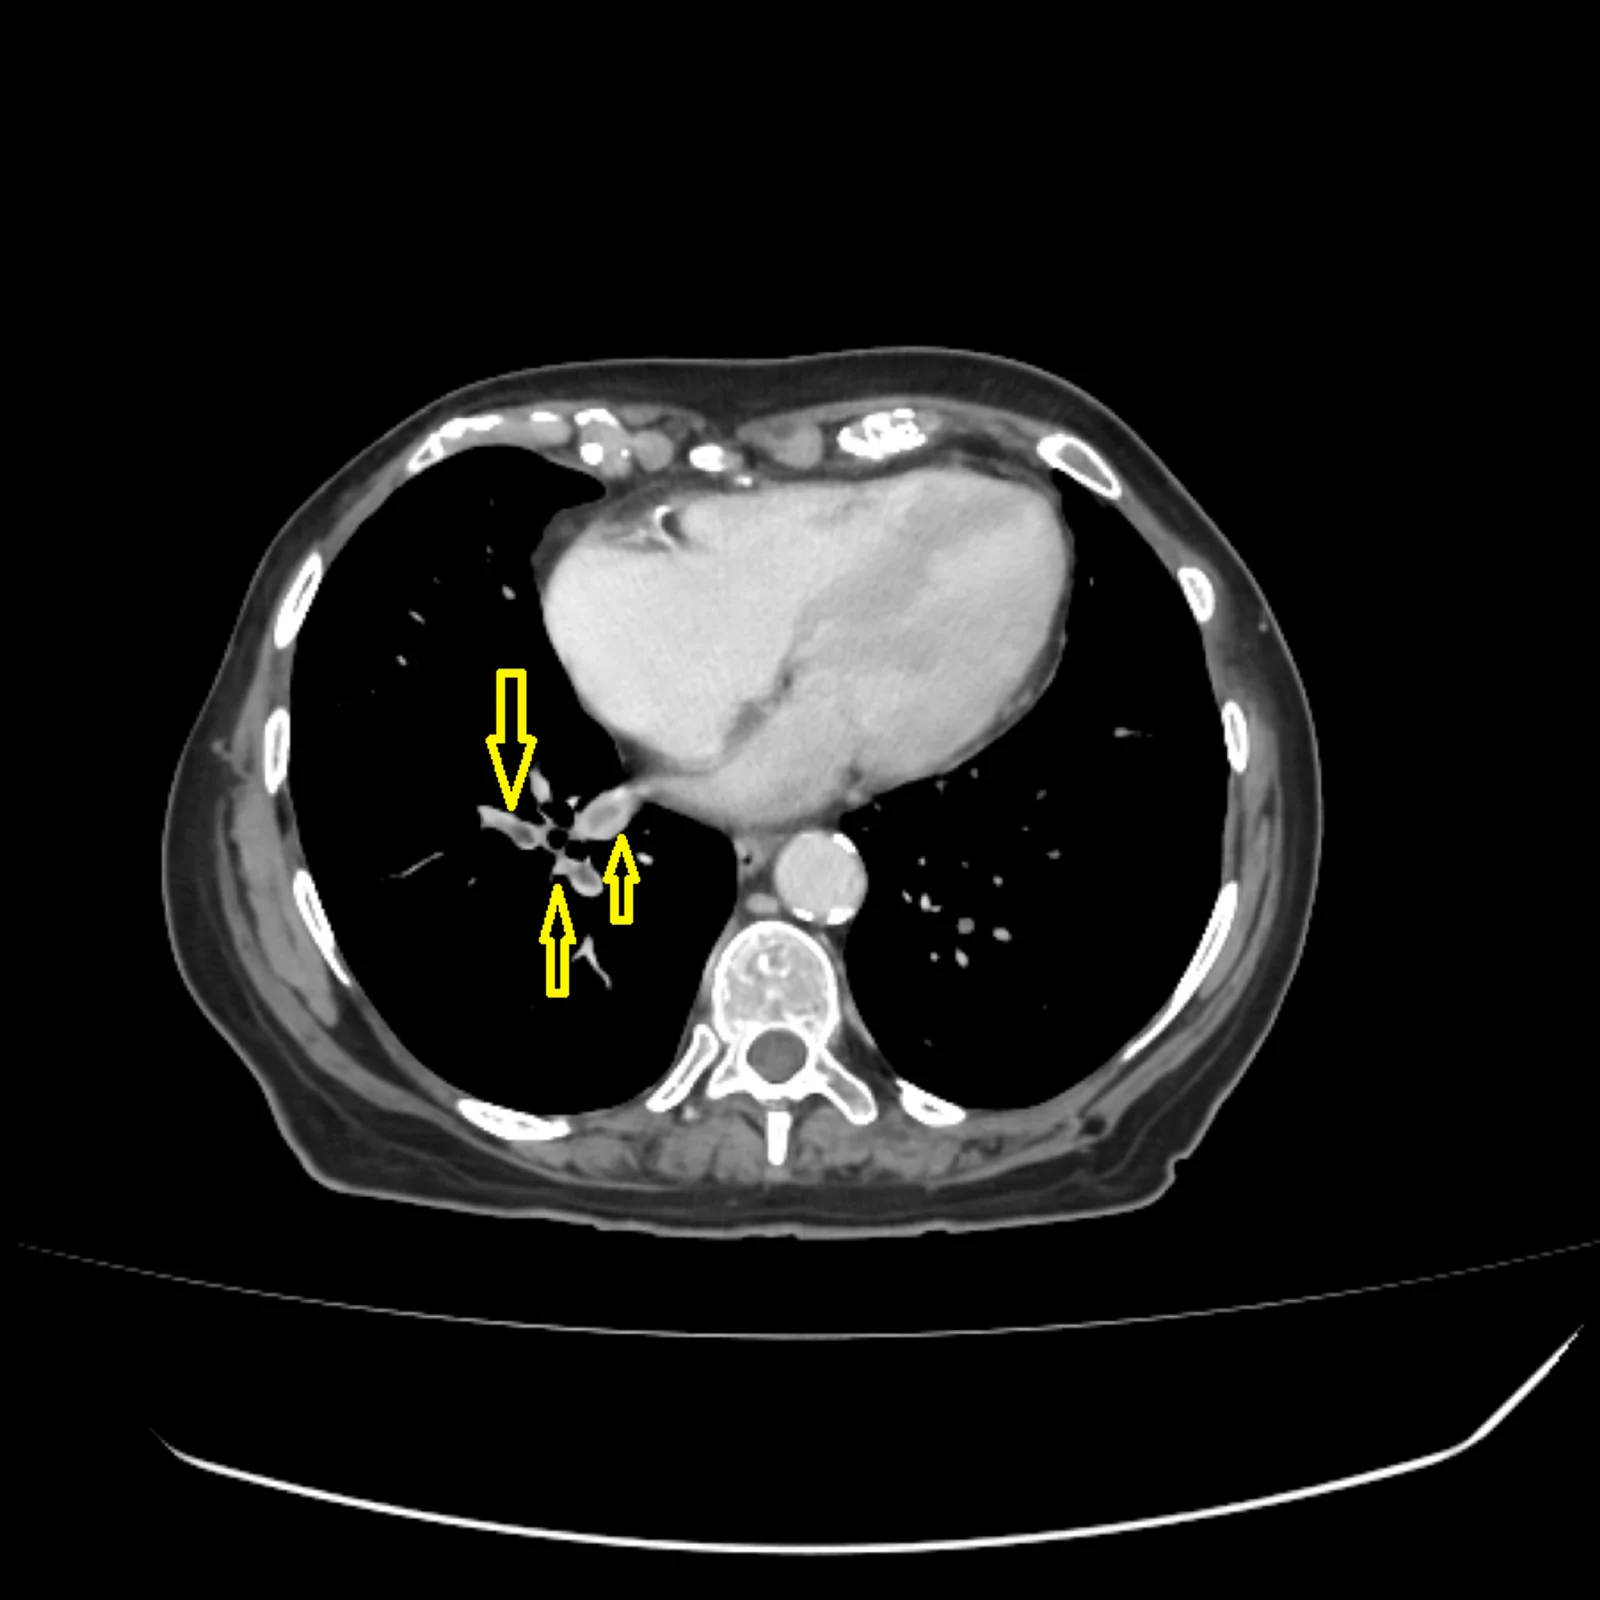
Cross-section of CT thorax with contrast, the yellow arrows pointing towards multiple small embolisms in pulmonary vasculature.
